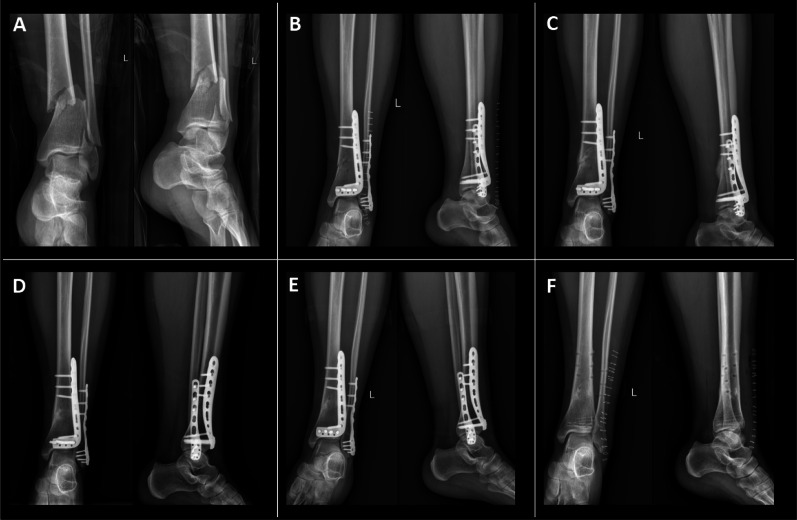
Patient with UDTF treated with DTP: A Left-sided ultra-distal tibia and fibula fractures; B Postoperative radiograph at 1 day; C Radiograph at 3 months postoperatively; D Radiograph at 6 months postoperatively; E Radiograph at 1 year postoperatively; F Radiograph following implant removal
